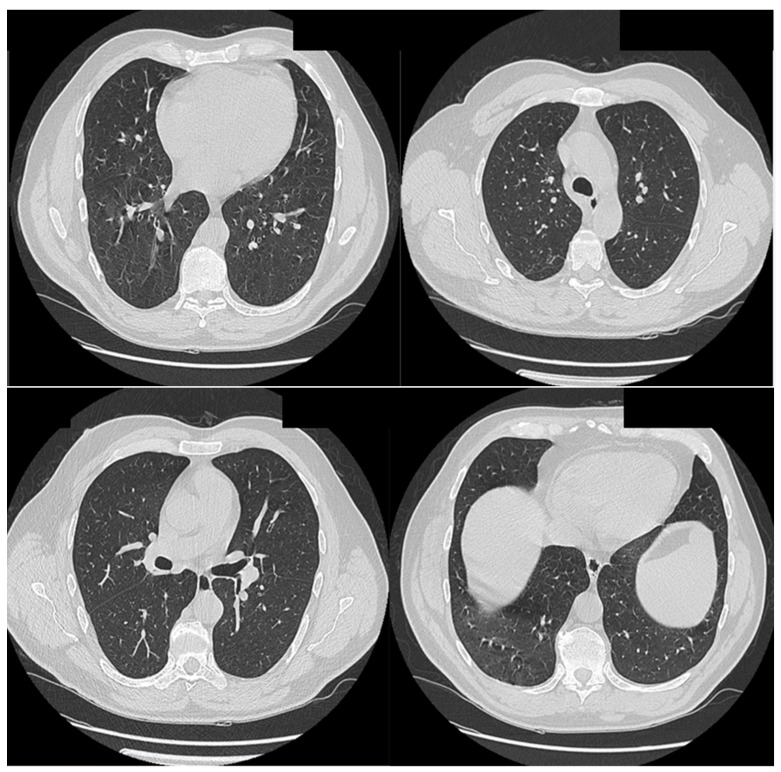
Thoracic CT (scale bar equals 1.25 mm). Fine fibrous band in the right posterior-basal region.
